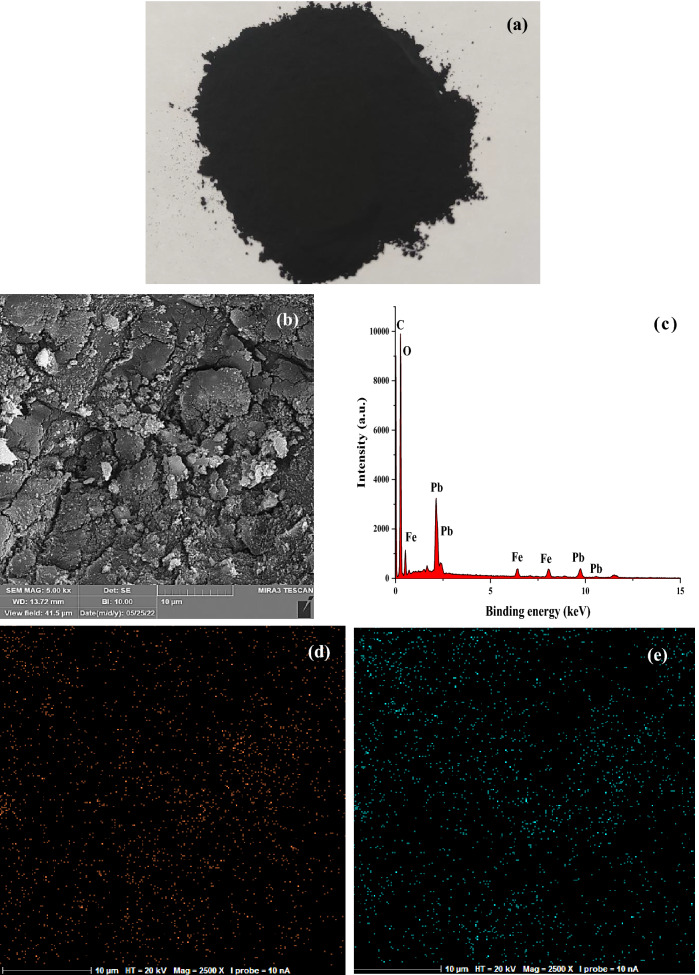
(a) Real picture, (b): SEM image, (c): EDX analysis of MSACadsorbent, and X-ray mapping of (d): lead, and (e): iron in MSAC.
